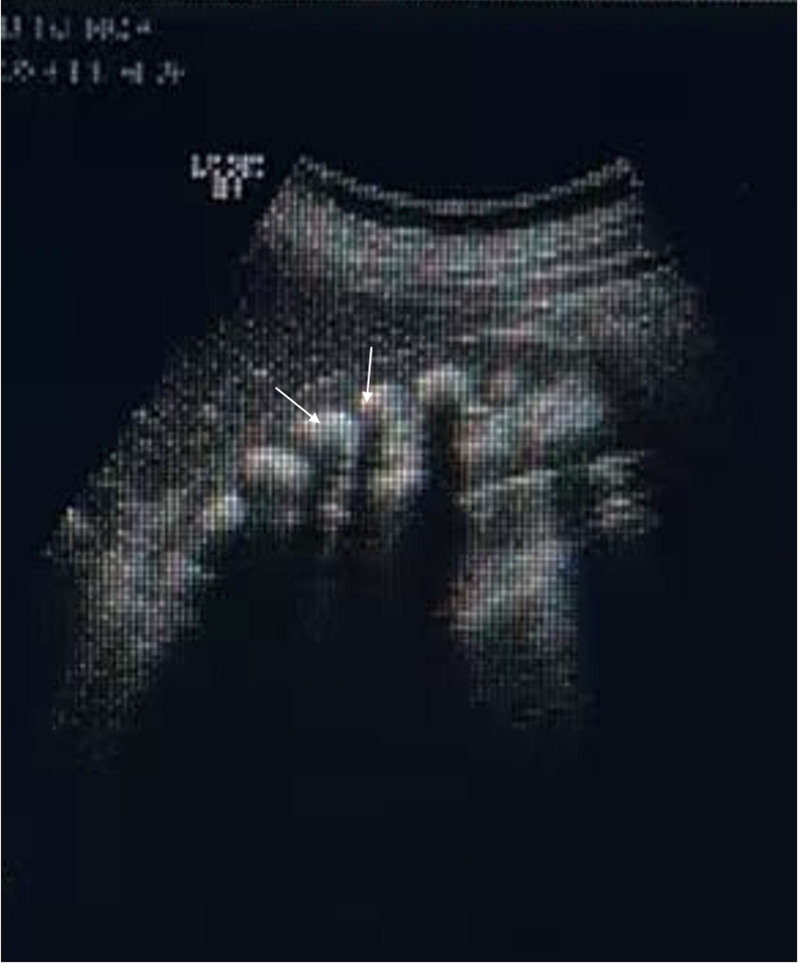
Sonography of kidneys. Strong echoes can be seen in diffuse pyramids of both kidneys, with larger ones measuring approximately 1.6 cm and accompanied by acoustic shadows.
